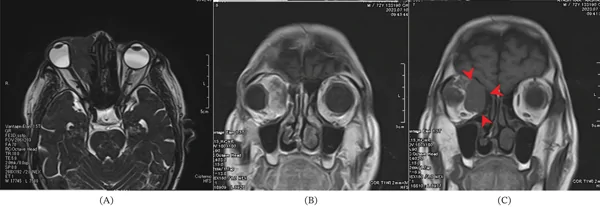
Preoperative MRI images of the patient in T1‐ and T2‐weighted sequences following gadolinium contrast administration. (A) Axial heavily T2‐weighted sequence MRI displaying a large, well‐defined, hypointense mass in the right orbit. (B) Coronal T1‐weighted noncontrast MRI showing the low‐signal intensity (hypointense) tumor in the superior‐medial right orbit. (C) Coronal T1‐weighted postcontrast (+IV gadolinium) MRI displaying heterogeneous enhancement of the mass. Red arrowheads delineate the multidirectional expansion of the mass, local tumor infiltration into the superior extraocular muscle complex, medial orbital wall, and lacrimal sac region.
